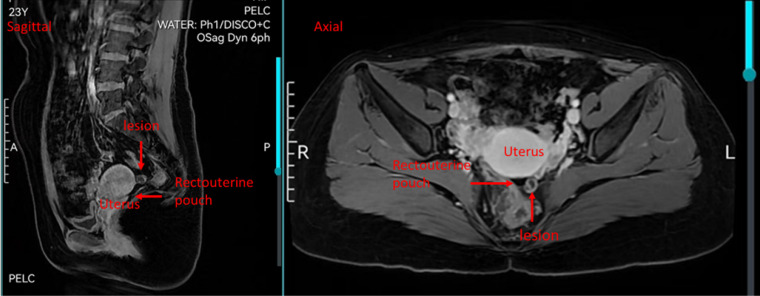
Pelvic magnetic resonance imaging (MRI) findings. Left: sagittal pelvic MRI showing a subtle lesion posterior to the uterus within the rectouterine pouch (pouch of Douglas) (arrow). Right: axial pelvic MRI demonstrating the corresponding posterior pelvic lesion (arrow). The lesion was more clearly appreciated on retrospective review of the MRI images.
